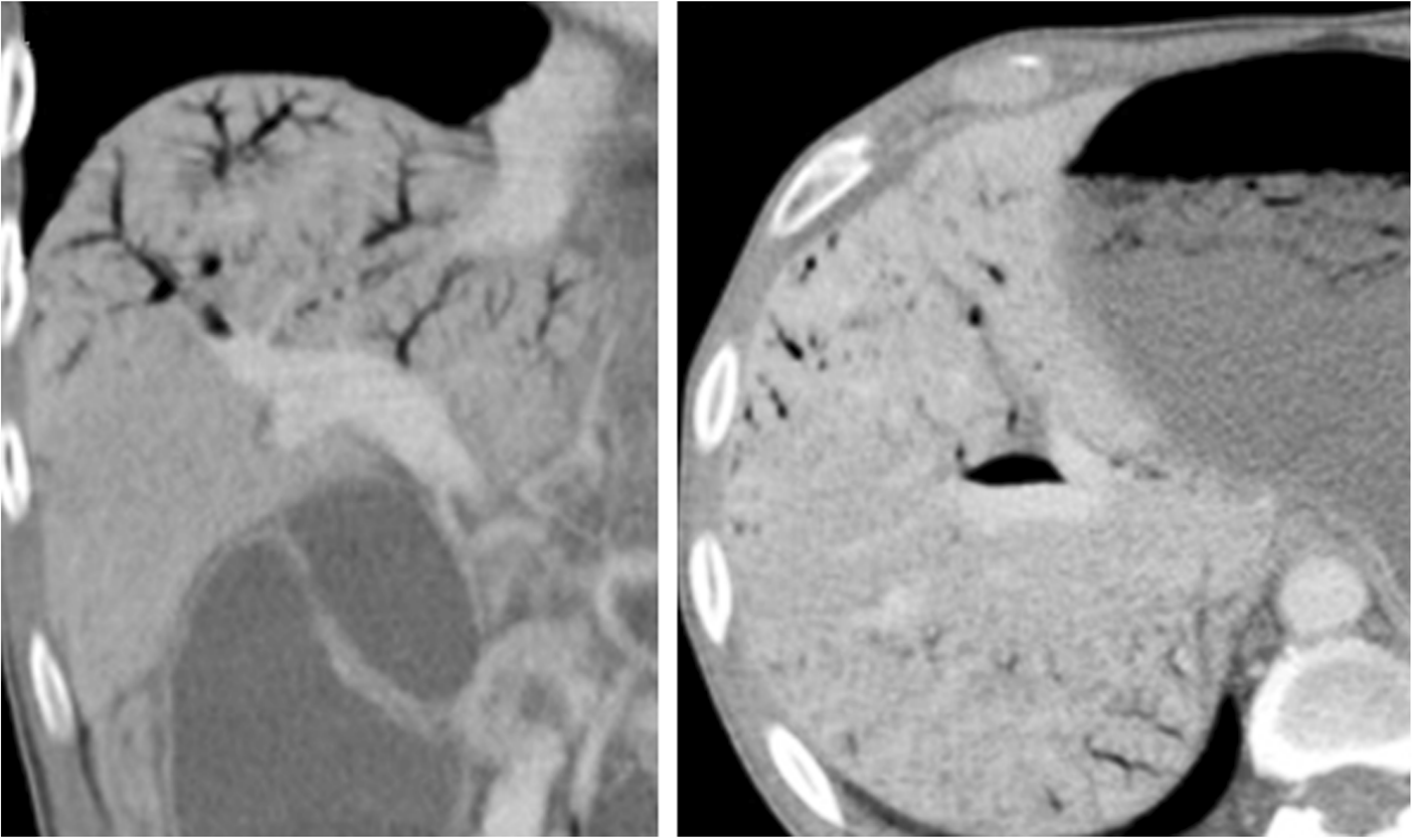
Portal venous gas. MPR images from portal venous phase CT shows air branching pattern fulfilling some segments of peripheral right PV branches
